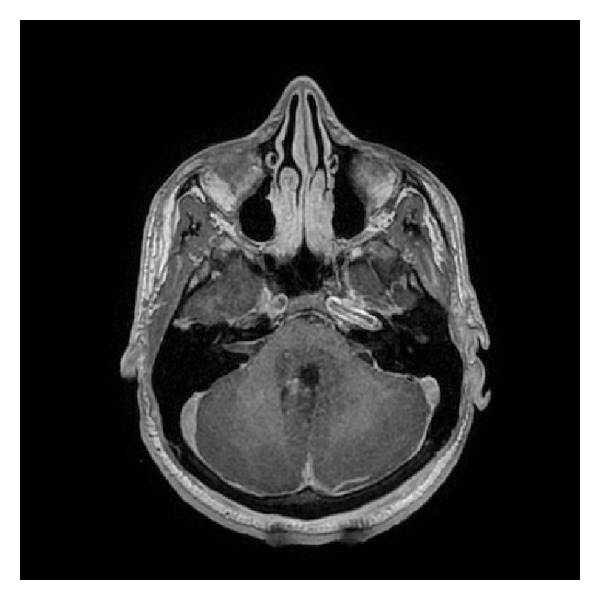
T1-weighted, immediate postoperative, gadolinium-enhanced axial magnetic resonance image, showing gross total resection with small enhancing changes along the posterior aspect of the surgical resection cavity.
